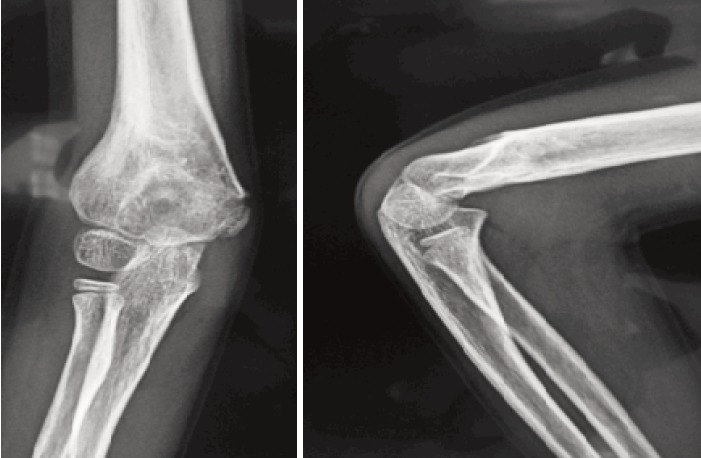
Final Follow-up radiographs of the patient.
